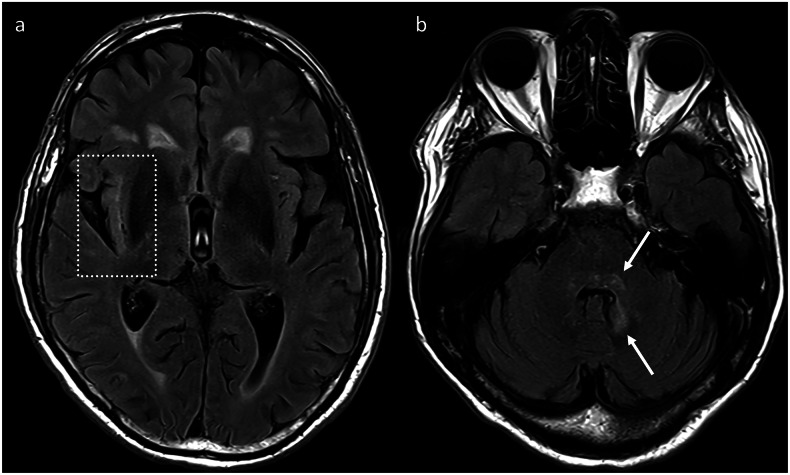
Axial FLAIR images in a patient with irAE-encephalitis demonstrating high signal in the right insula (a, dotted square), as well as in the dorsal pons extending to the adjacent left cerebellar hemisphere (b, arrows). The patient had initially presented with progressive unsteadiness after 4 cycles of cemiplimab for metastatic cutaneous squamous cell carcinoma. CSF analysis demonstrated a lymphocytosis, without any neuronal antibodies
